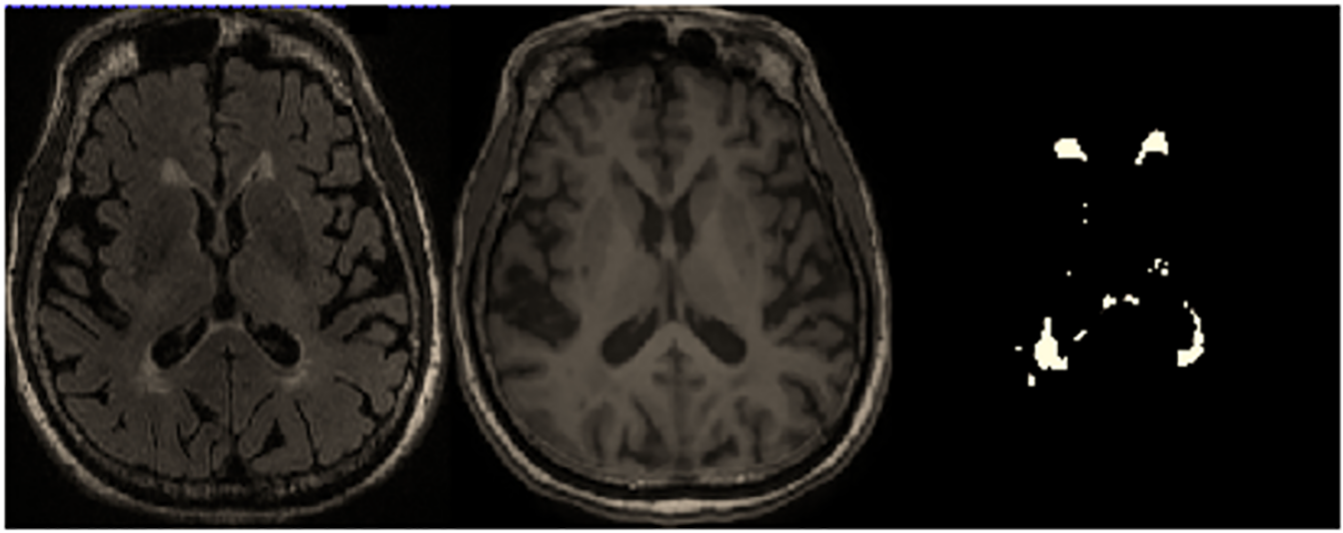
The VBM analysis. Axial T2-Weighted FLAIR Image, T1-Weighted FSPGR Image and lesions segmented using LST of SPM8.
